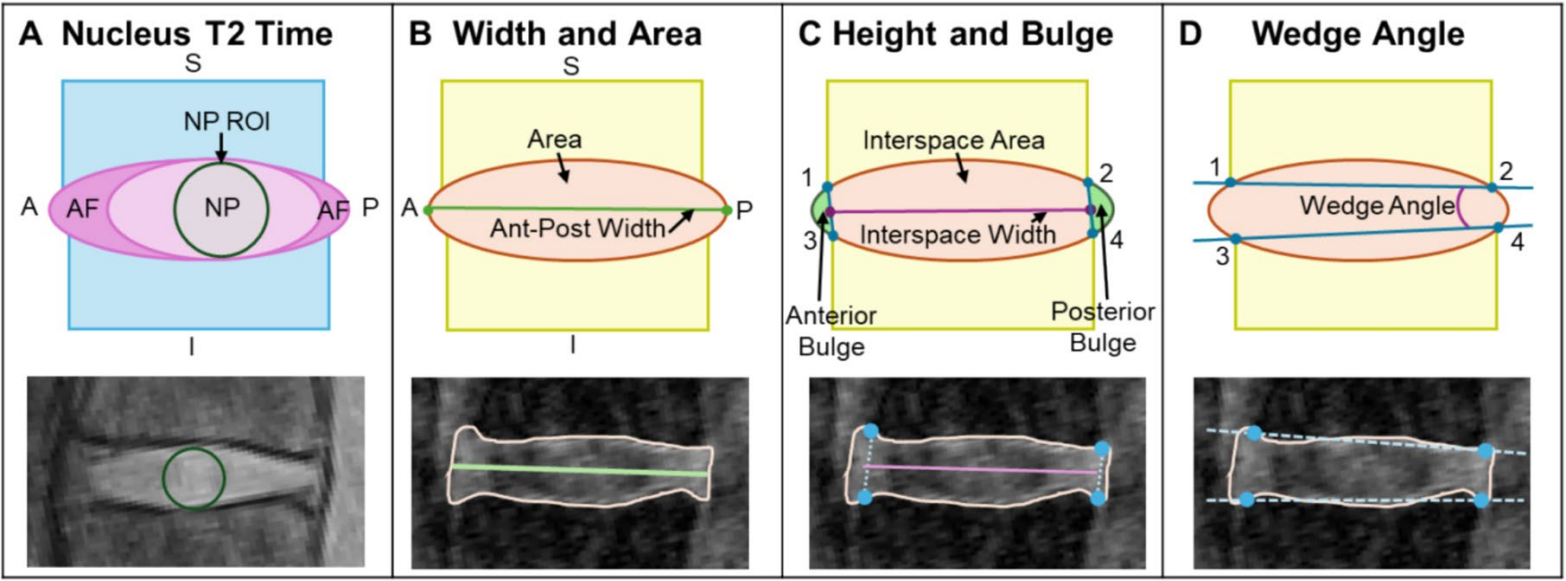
MRI outcome measures. A T2 relaxation time was calculated from the nucleus pulposus region of interest (NP ROI). Disc geometry was quantified by B disc mid-sagittal area, anterior–posterior width, C disc height, anterior and posterior disc bulge, and D disc wedge angle
